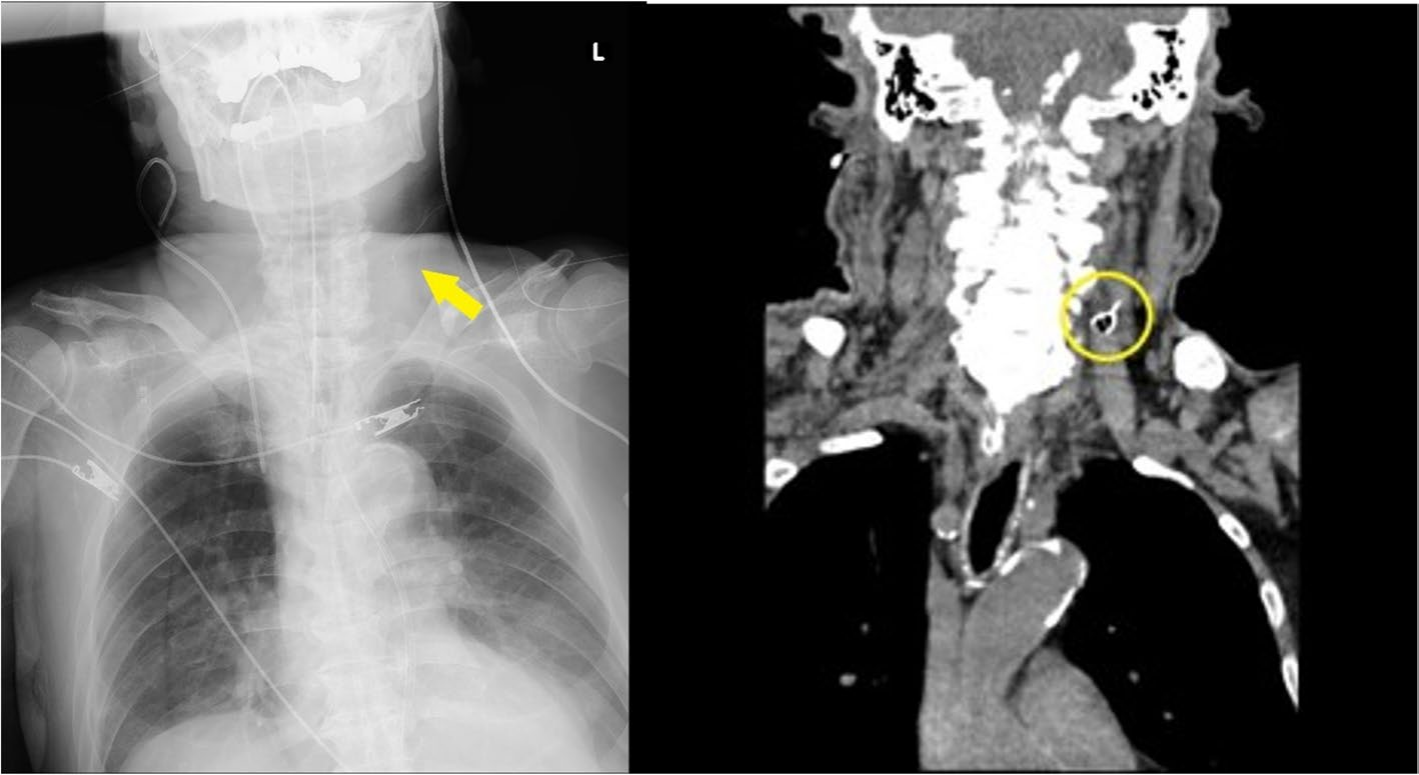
Chest radiograph (left) in the anteroposterior view, showing the guidewire looped in the neck (yellow arrow), and computed tomography of the neck (right), showing the tangled guidewire (yellow circle) near the left internal jugular vein
